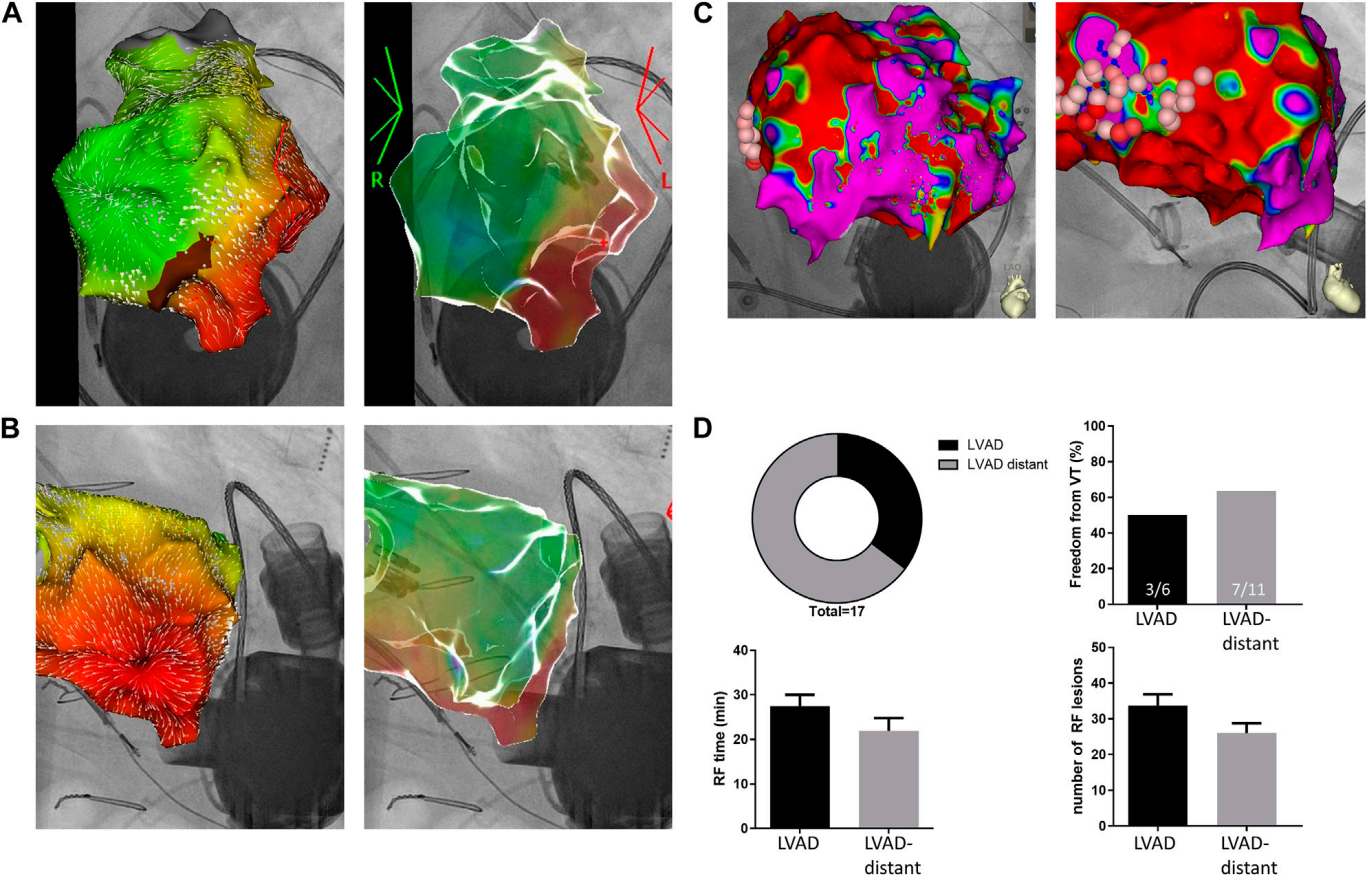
(A and B). LVAD related VT: Anterior-posterior AP; (A) and left anterior oblique LAO; (B) view of the left ventricle. CARTOUNIVU overlay of 3D electroanatomic map [local activation time and carto-confidence (white arrows) map] and X-ray image. Mapping was performed during an ongoing focal VT from the LVAD insertion site. (C). Non-LVAD related VT patient: AP (left) and LAO (right) view of the left ventricle. CARTOUNIVU overlay of 3D electroanatomic map (bipolar voltage map; red areas <1.5 mV) with marked ablation sites distant from the LVAD cannula. (D). Distribution of LVAD associated and non-LVAD associated VT in LVAD patients and freedom from VT (top). Time of radiofrequency ablation (RF time) as well as number of radiofrequency ablation lesions (bottom). Patients had either an LVAD with LVAD-related or non-LVAD related VTs (LVAD-distant).
